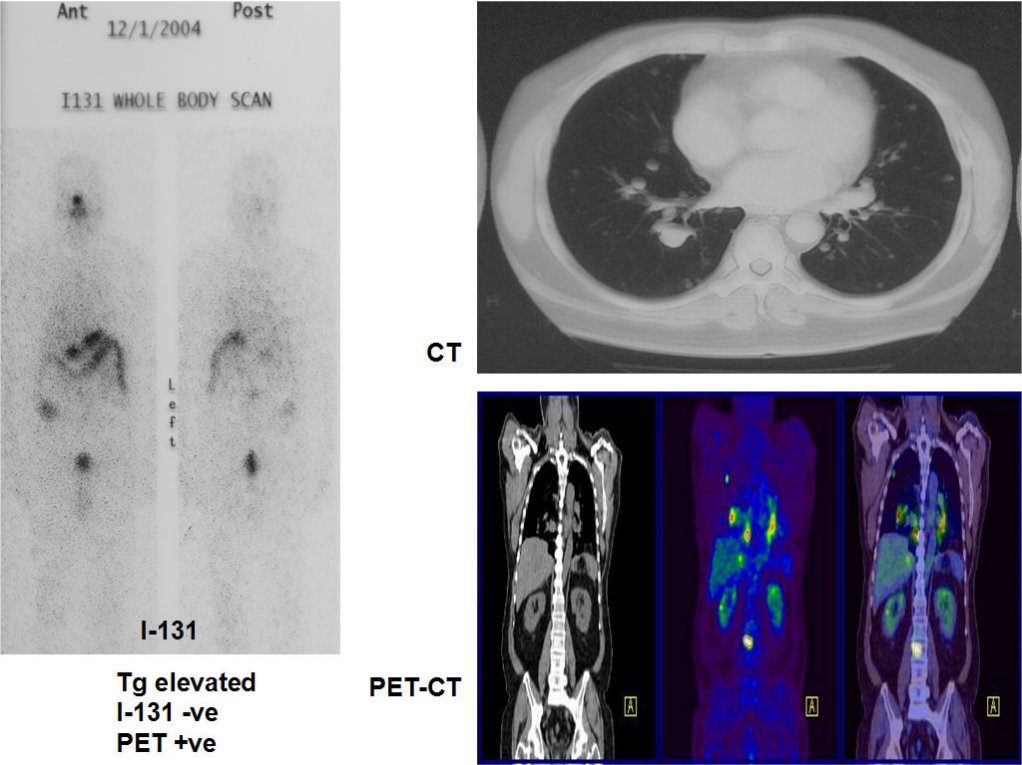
Negative I-131 scan in patient with lung metastasis on CT and PET scans.
